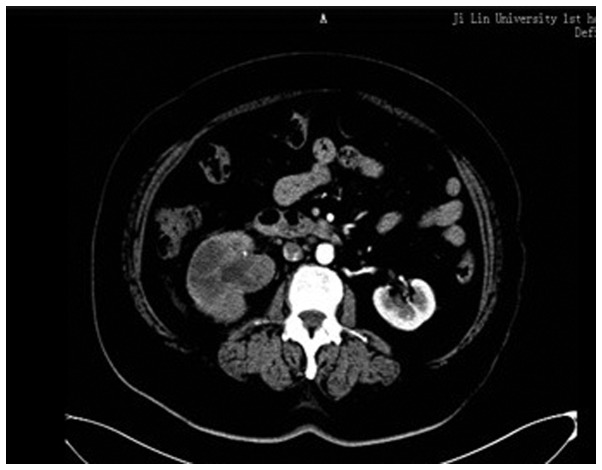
CT scan showing a well-defined 2–3-cm mass invading the right renal pelvis. The mass shows poor contrast enhancement.
